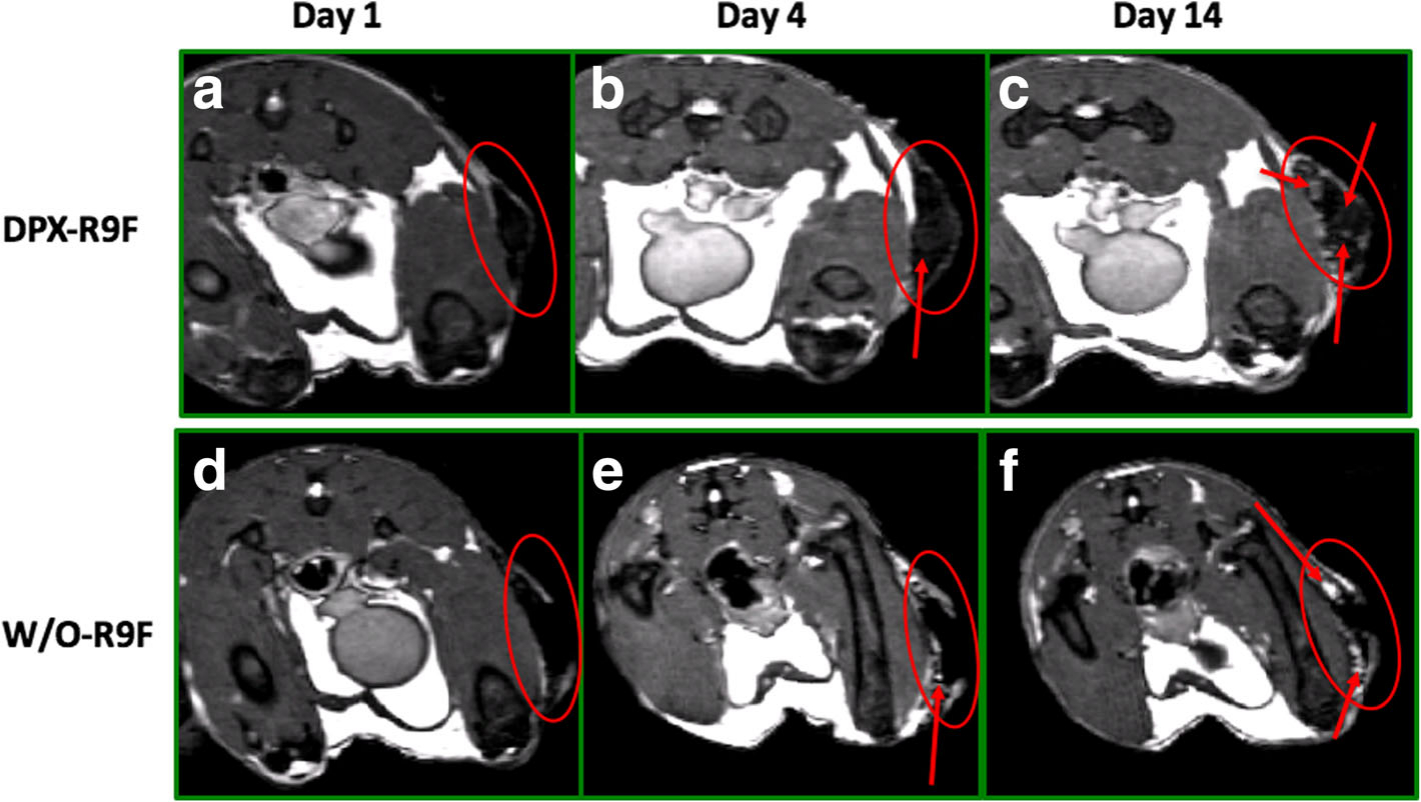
Clearance of Antigen in DPX-R9F and w/o-R9F Detected by MRI. MR images displaying iron content of SPIO-R9F in either a), b) and c) DPX-R9F or d), e) and f) w/o-emulsion. Images were acquired one (a & d), four (b & e), or fourteen (c & f) days post-immunization. In DPX-R9F SPIO-antigen clears centrally from the vaccine site, from the middle of the site of injection (SOI) outwards, while the SPIO-R9F clears from the edges of the w/o-R9F depot. Red arrows indicate site of active clearance
